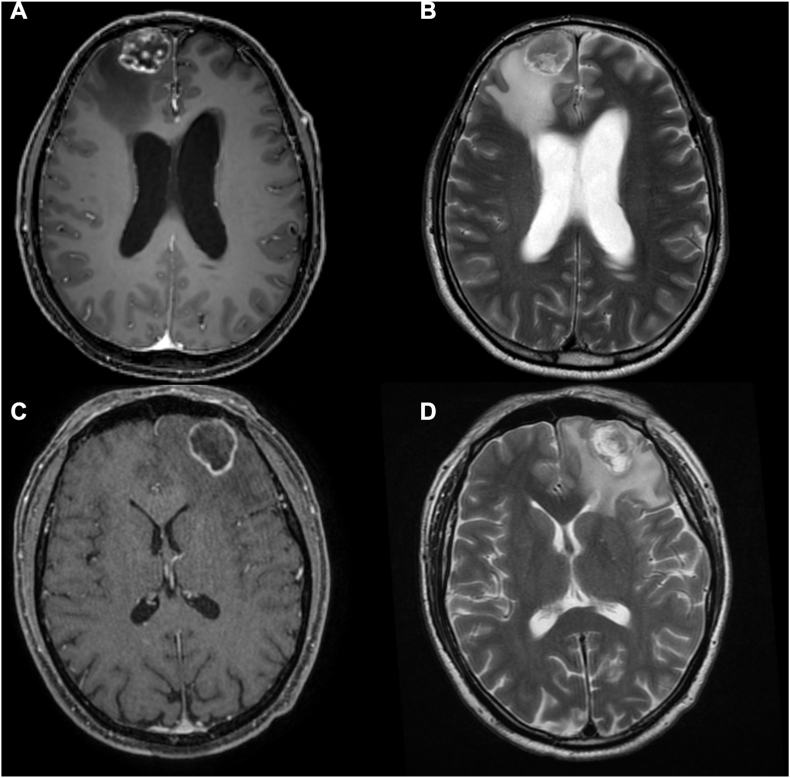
The image shows MRI images of two patients with frontal brain metastasis. In case A, the breast cancer patient has a less edemigenous solid metastasis than the patient in case B with NSCLC, who has a cystic mass with diffuse edema.
